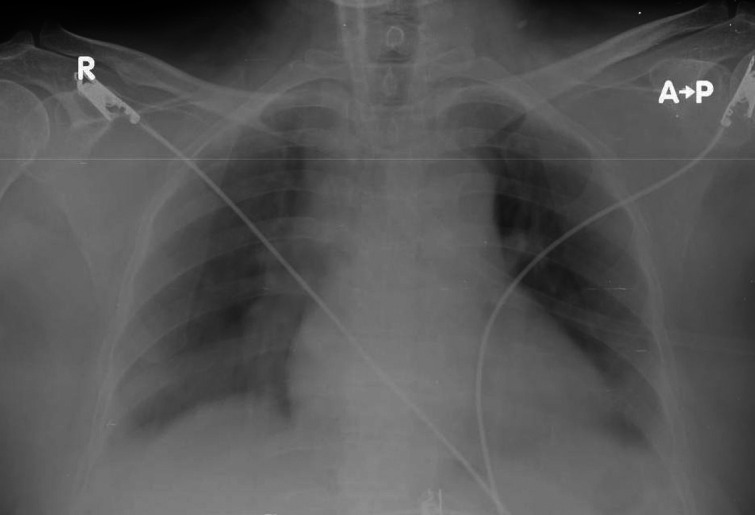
Preoperative chest x-ray showing features of pneumonia.
